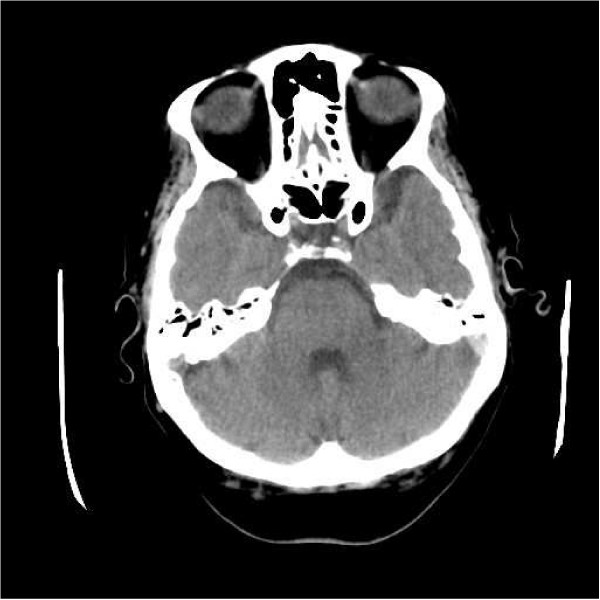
Axial section computed tomography scan demonstrating hyperdensity in the left sigmoid sinus.
